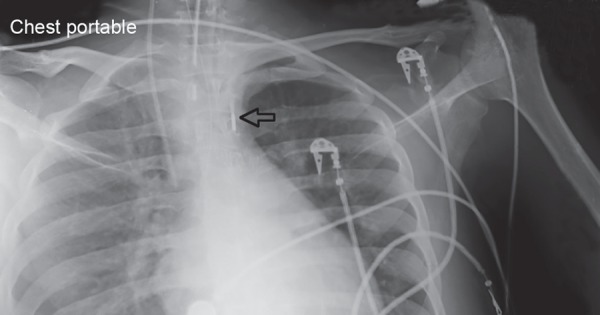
X-ray chest of patient on day 6. Intra-aortic balloon pump can be seen just distal to arch of aorta. Clearing of pulmonary edema as compared to the previous X-ray can be noted.
